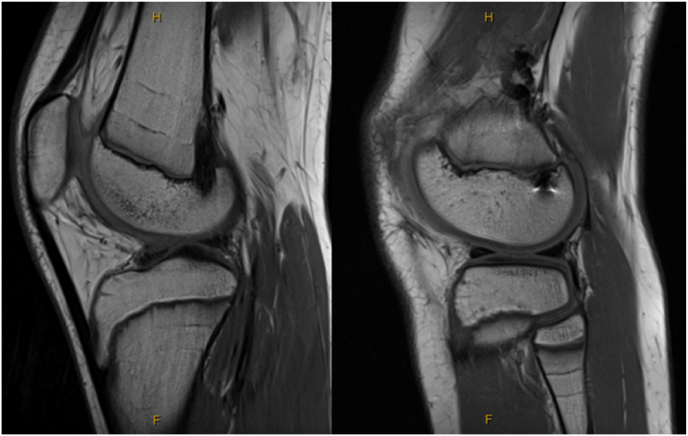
Skeletally immature patient post operative MRI image showing Harris growth arrest lines.
